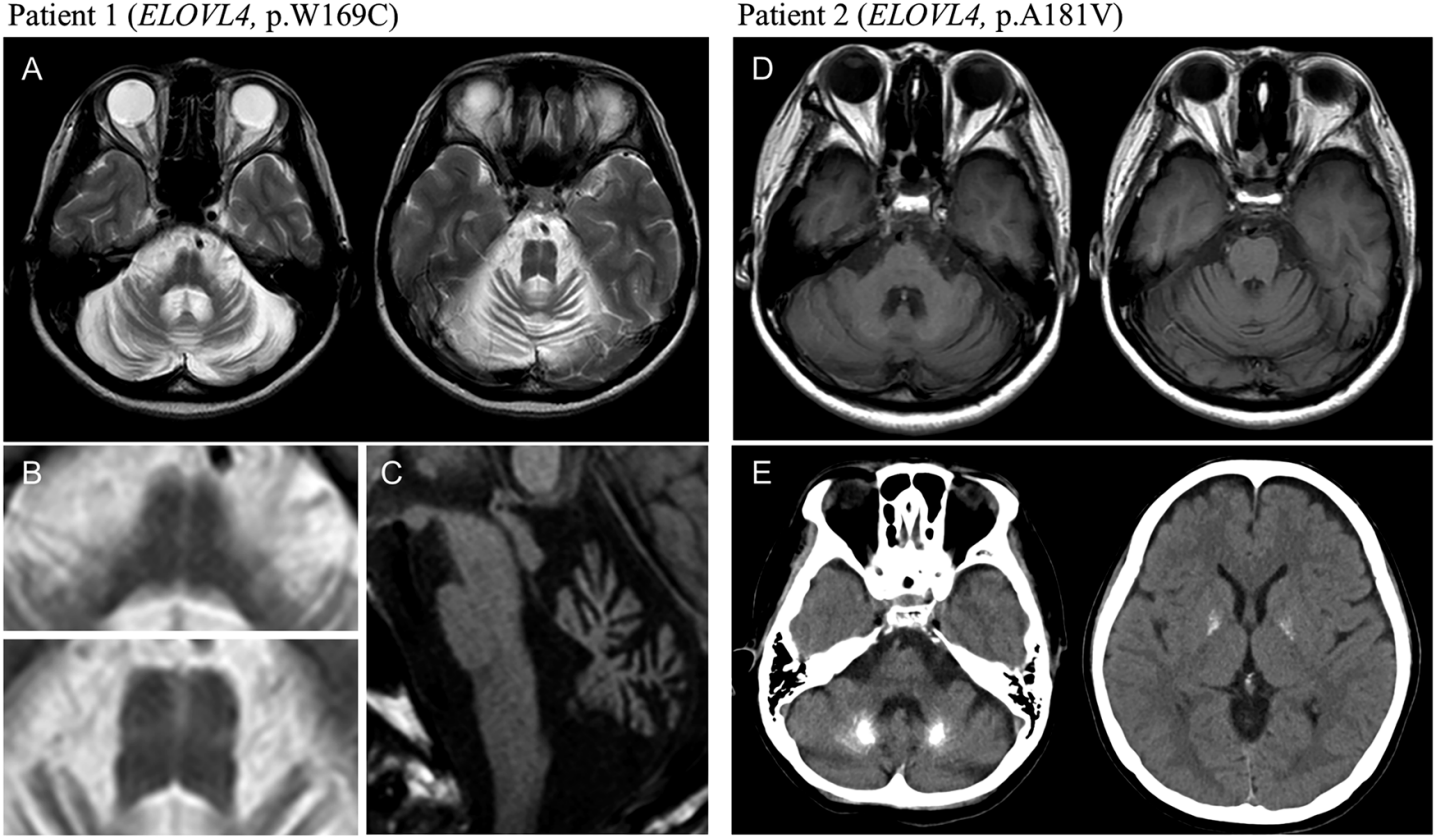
Head images of the patients with *ELOVL4* variants. A Axial T2-weighted images of Patient 1 show cerebellar and brainstem atrophy as well as the hot cross bun sign in the pons. B The hot cross bun sign is clearly observed in the enlarged images of the pons in (A). C Sagittal T1-weighted image of Patient 1 showing the cerebellar vermis and brainstem atrophy. D Axial T1-weighted images of Patient 2, showing mild cerebellar and brainstem atrophy. E Head computed tomography of Patient 2 showing calcifications in the globus pallidus and dentate nucleus
